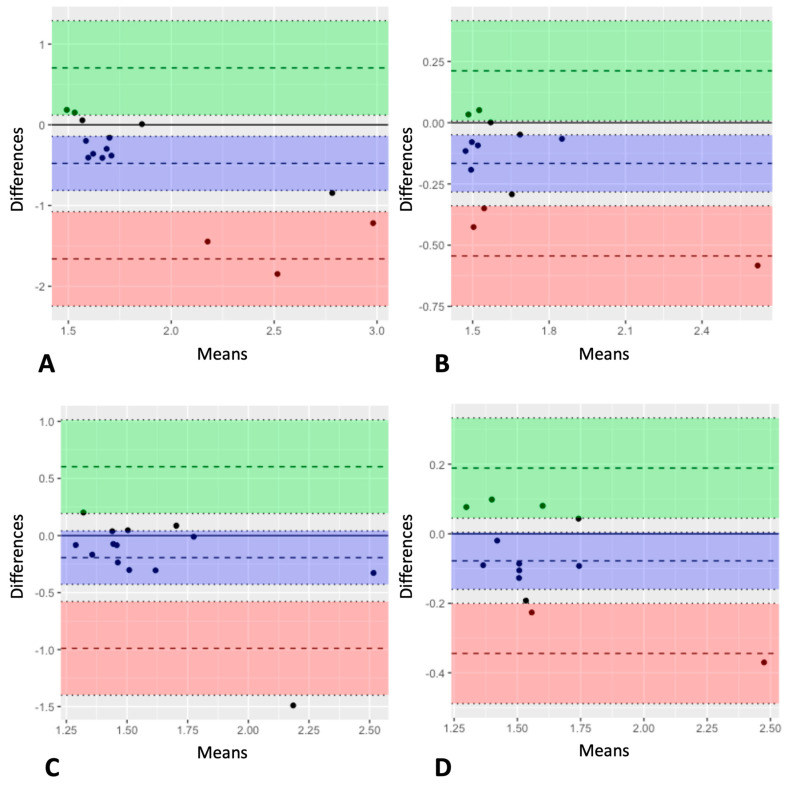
Bland–Altman plots for comparison of the two methods: shear wave speed measurements using MRE vs. SWE. *x*-axis: mean of MRI and US measurements; *y*-axis: difference between MRE and SWE (negative values indicate higher SWE-derived stiffness compared with MRE values). Horizontal lines are placed at both the mean difference and the agreement boundaries, which were calculated by adding and subtracting 1.96 times the standard deviation of the differences from the mean difference. In general, SWE yielded higher stiffness values. (A) Baseline, *n* = 15; (B) EOT, *n* = 13; (C) EOT+3, *n* = 14; and (D) EOT+12, *n* = 13.
